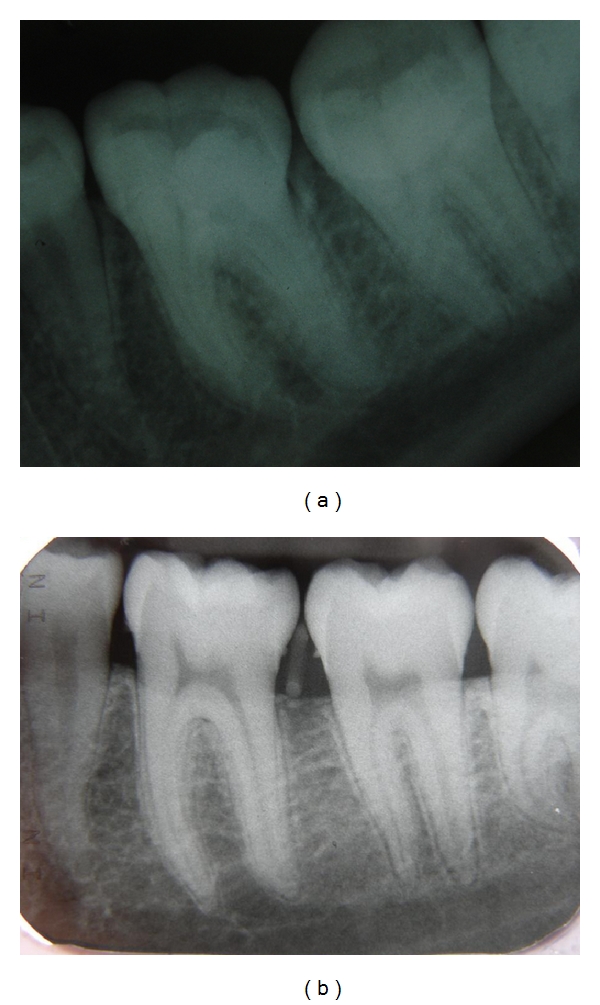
(a) Radiographic view of the elastic rubber band between the first and second lower left molars. (b) A proper periapical X-ray revealing an elastic band in the periodontal space.
